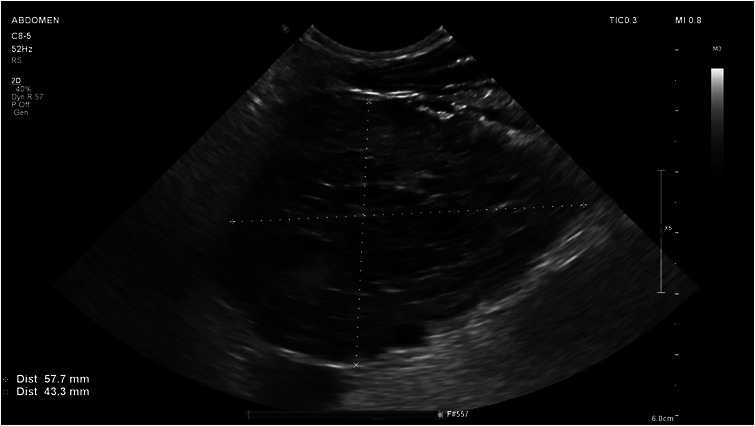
Abdominal ultrasonography at initial diagnosis. (a) Transverse view showing a large heterogeneous hypoechoic mass (5.8 × 4.3 cm) with poorly defined margins. (b) Longitudinal view adjacent to the right kidney demonstrating the same heterogeneous mass, with a small amount of peritoneal effusion.
